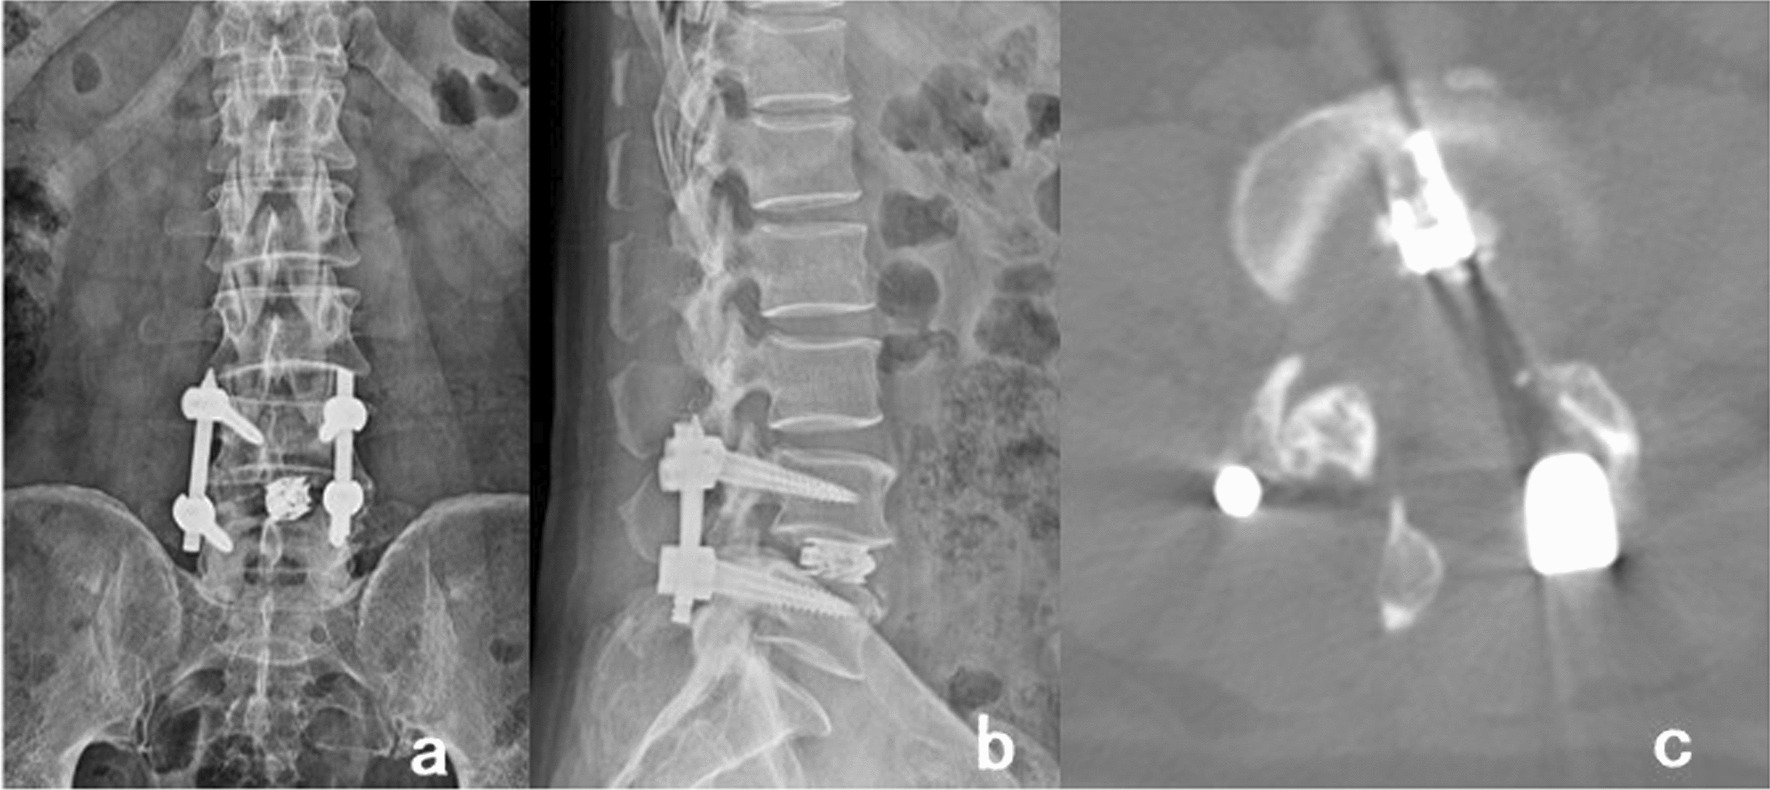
a, b Post-operative anteroposterior and lateral X-ray radiographs showed the pedicle screws, and the cage were in good position; c Post-operative CT in coronal position showed that the cage was in good position, and the cross-sectional area of the spinal canal was significantly enlarged compared with that before operation
